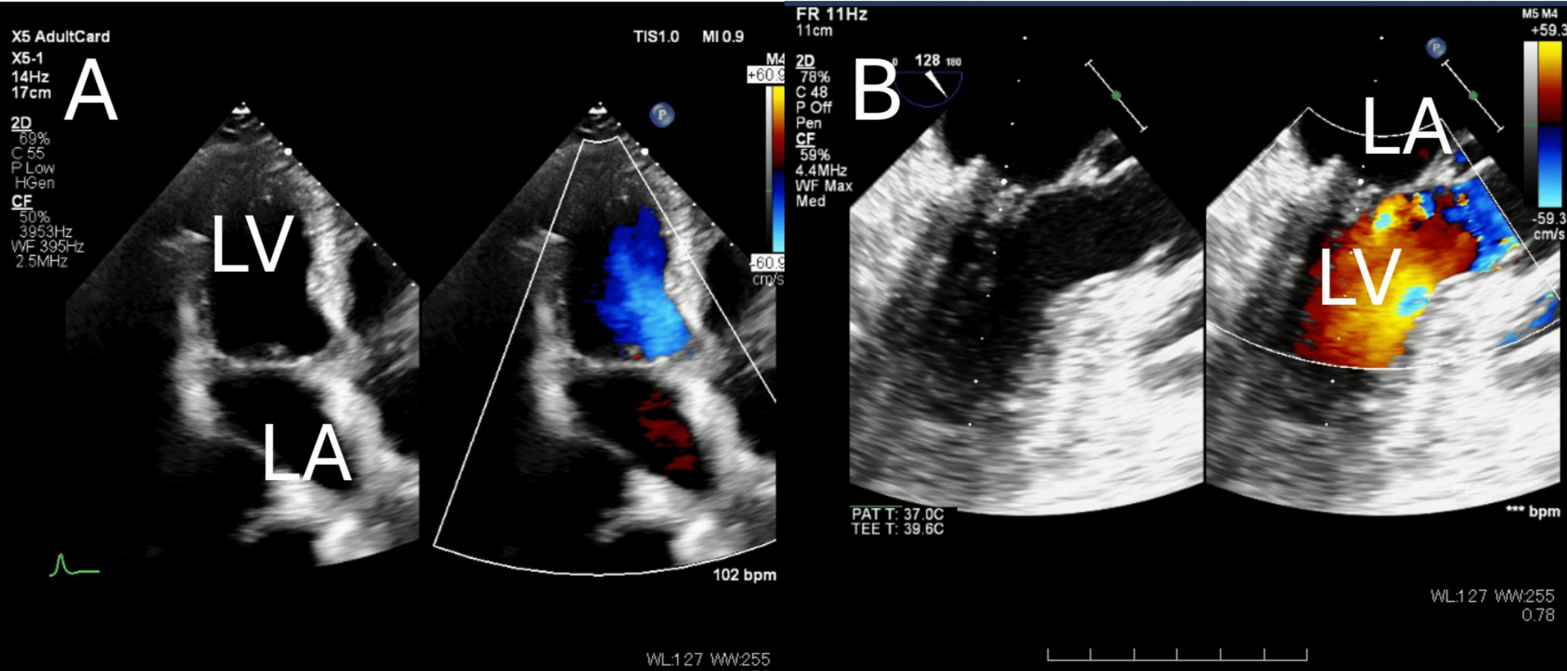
Postoperative echocardiographic evaluation. (A) Transthoracic echocardiography (TTE) after surgery demonstrates no residual mitral regurgitation. (B) Intraoperative transesophageal echocardiography (TEE) following repair also confirms the complete elimination of regurgitation.
